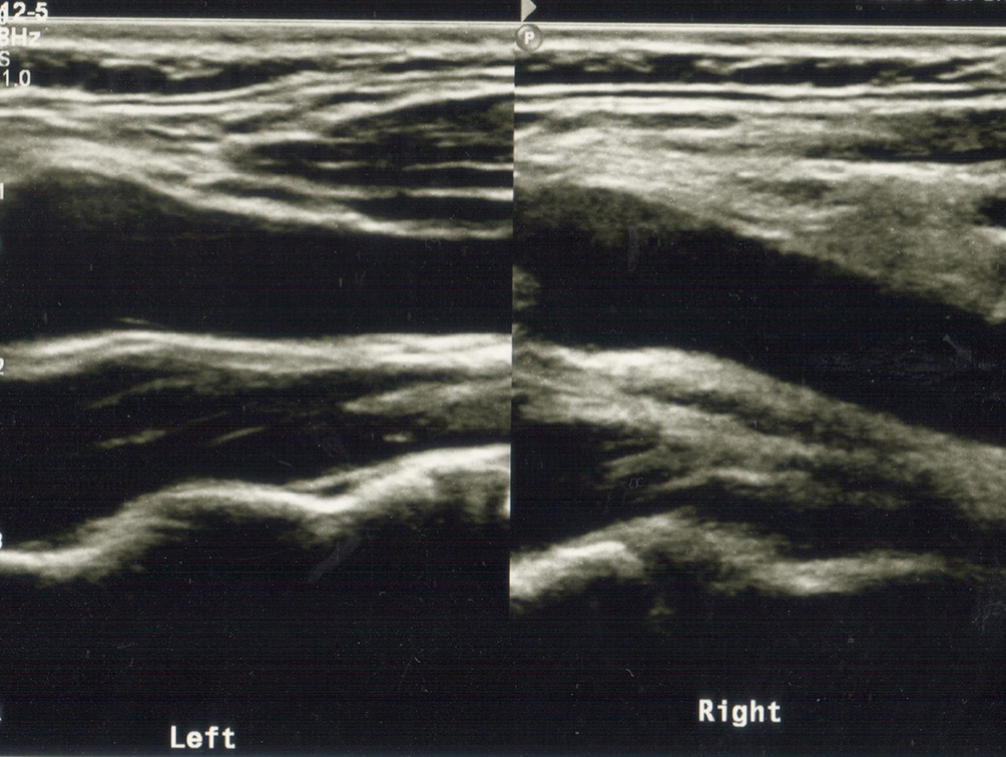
Ultrasonogram of the right side of the neck showing increased echogenicity in the perivascular tissue of the distal common carotid artery and the carotid bulb region with obliteration of normal tissue planes. Normal sonographic appearances of the left carotid artery (left side of the image).
